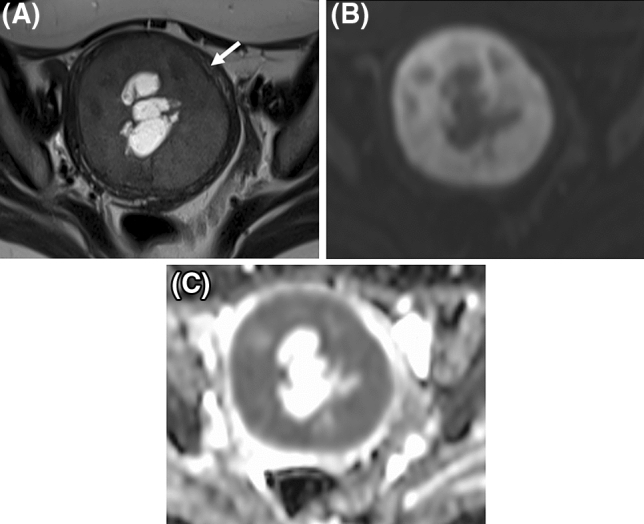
A representative case of low grade endometrial stromal sarcoma with surrounding low signal intensity (SI) rim on T2-weighted images (A, arrow). Cystic/necrotic change is also observed. Solid parts of tumor shows high SI on diffusion-weighted images (B) with low apparent diffusion coefficient values (C).
